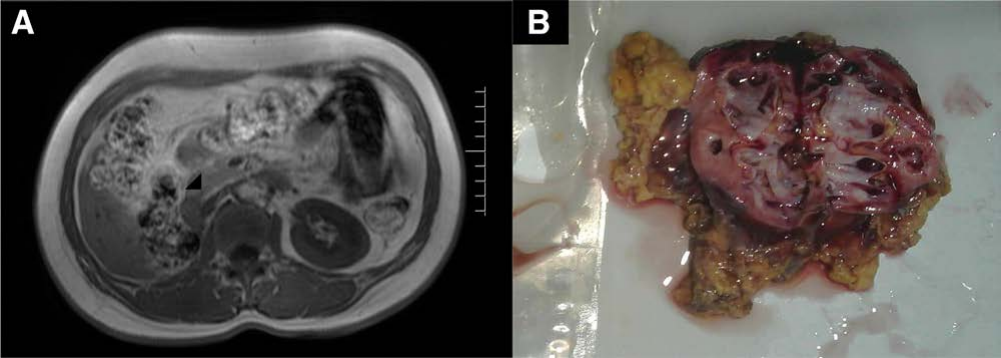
CT and macroscopic. (A) CT shows significant atrophy of the right kidney (arrow). (B) Gross image shows atrophy of the right renal parenchyma, with dilation of the renal pelvis and calyces. CT = computed tomography.
